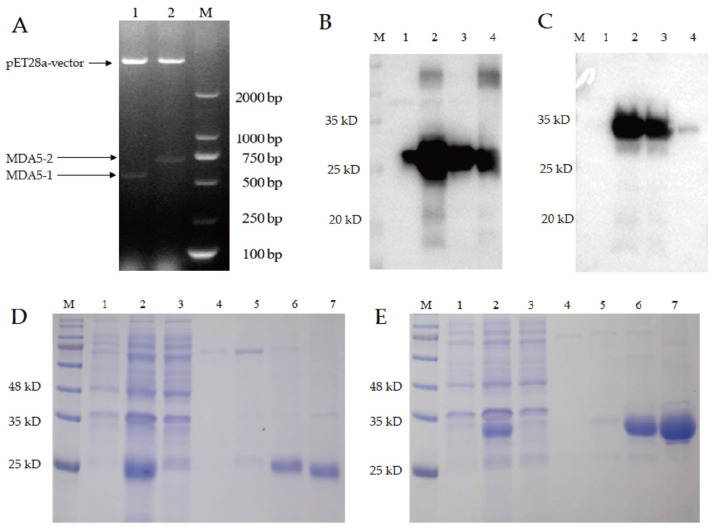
Construction of prokaryotic expression vector and expression identification of MDA5-1 and MDA5-2 proteins. (A) Identification of recombinant plasmids pET28a-His-MDA5-1 and pET28a-His-MDA5-2 via enzyme double digestion. (B) Identification of recombinant MDA5-1 protein via Western blotting. (C) Identification of recombinant MDA5-2 protein via Western blotting: 1—bacteria with recombinant plasmid before induction; 2—bacteria with recombinant plasmid after induction; 3—supernatant after ultrasound; 4—precipitation after ultrasound. (D) Purification of MDA5-1 protein. (E) Purification of MDA5-2 protein: 1—bacteria with recombinant plasmid before induction; 2—bacteria with recombinant plasmid after induction; 3—supernatant after filtration; 4–5—washing protein; 6–7—elution protein with 400 and 200 mM imidazole.
